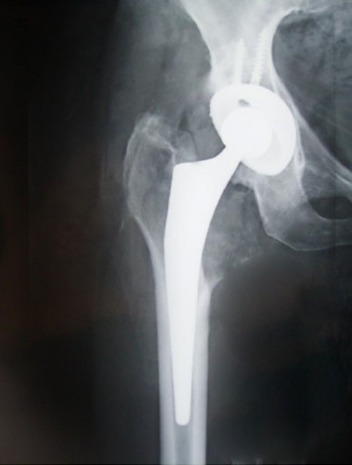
Radiographie de la hanche droite de face après arthroplastie
